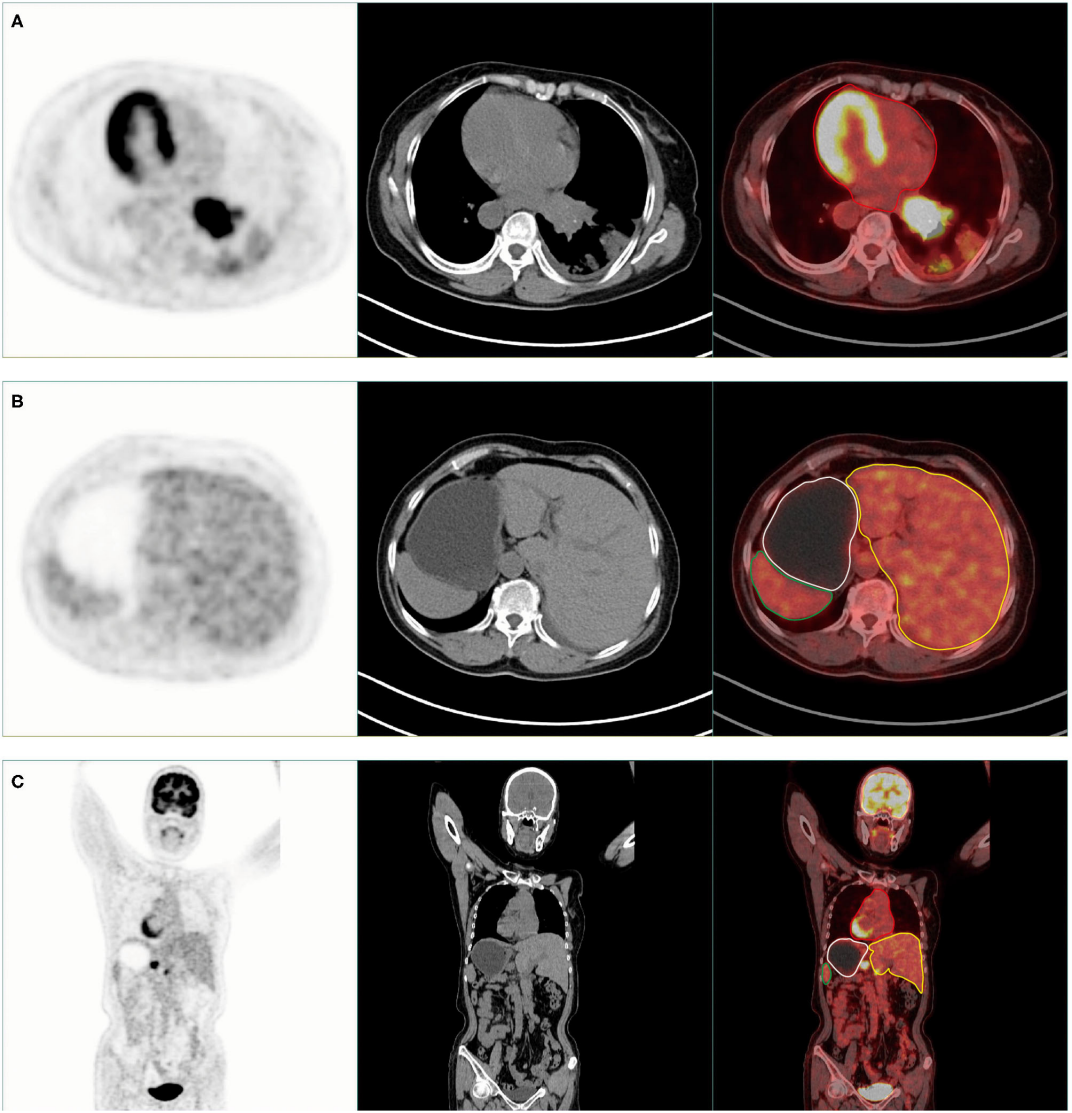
PET/CT scan showed that this was a patient with situs inversus totalis (SIT). (A,B) Axial PET, CT, and PET/CT fusion images show the dextrocardia (red area), liver on the left side (yellow area), stomach (white area), and spleen (green area) on the right side of the patient's body. (C) Coronal PET, CT, and PET/CT fusion images show the dextrocardia (red area), liver on the left side (yellow area), stomach (white area), and spleen (green area) on the right side of the patient's body.
